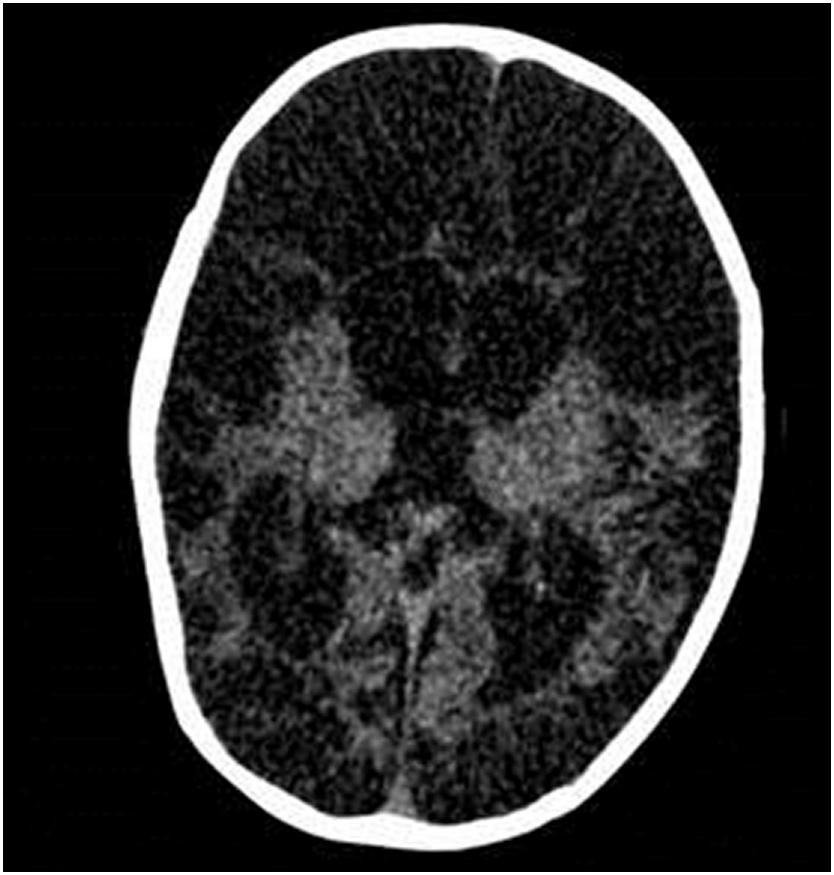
CT scan of patient #6 CT scan of patient #6 found an almost complete destruction of the parenchyma with only persistence of a thin cortical ribbon (resulting in a very large porencephalic cyst).
